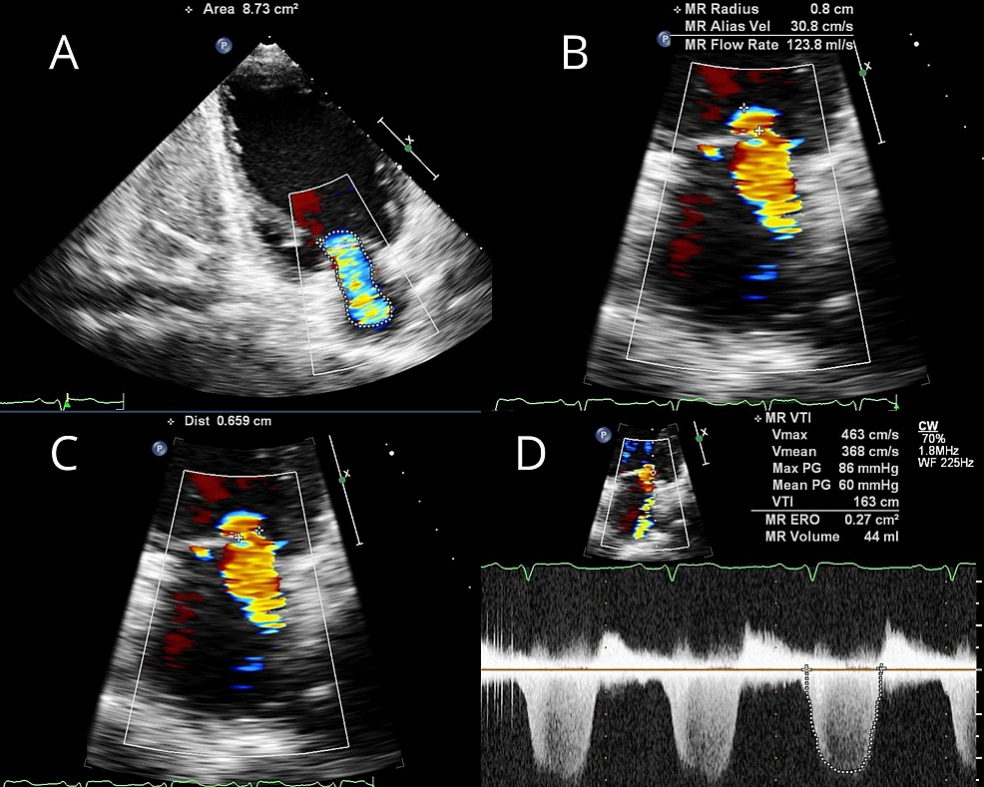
Transthoracic echocardiogram of mitral regurgitation (A) Two-chamber view showing mitral regurgitation with jet area 8.73 cm^2^. (B) Four-chamber view showing proximal isovelocity surface area of 0.8 cm. (C) Four-chamber view showing vena contracta of 0.659 cm. (D) Four-chamber view with continuous-wave Doppler showing effective regurgitant orifice area of 0.27 cm^2^.
